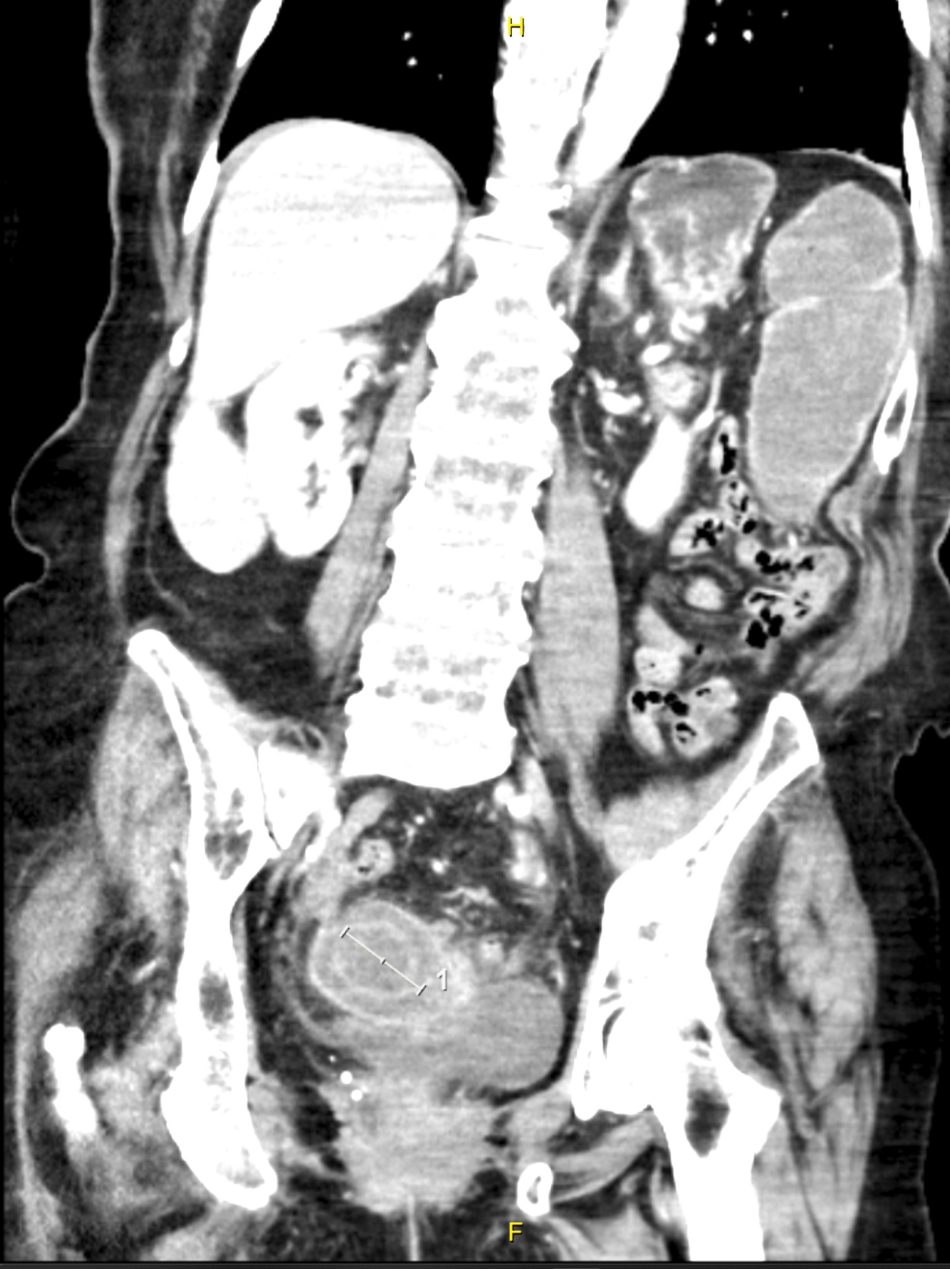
Abdominal CT scan: axial view showing a 34.74mm × 34.97mm gallbladder stone impacted in the mid to distal sigmoid colon
